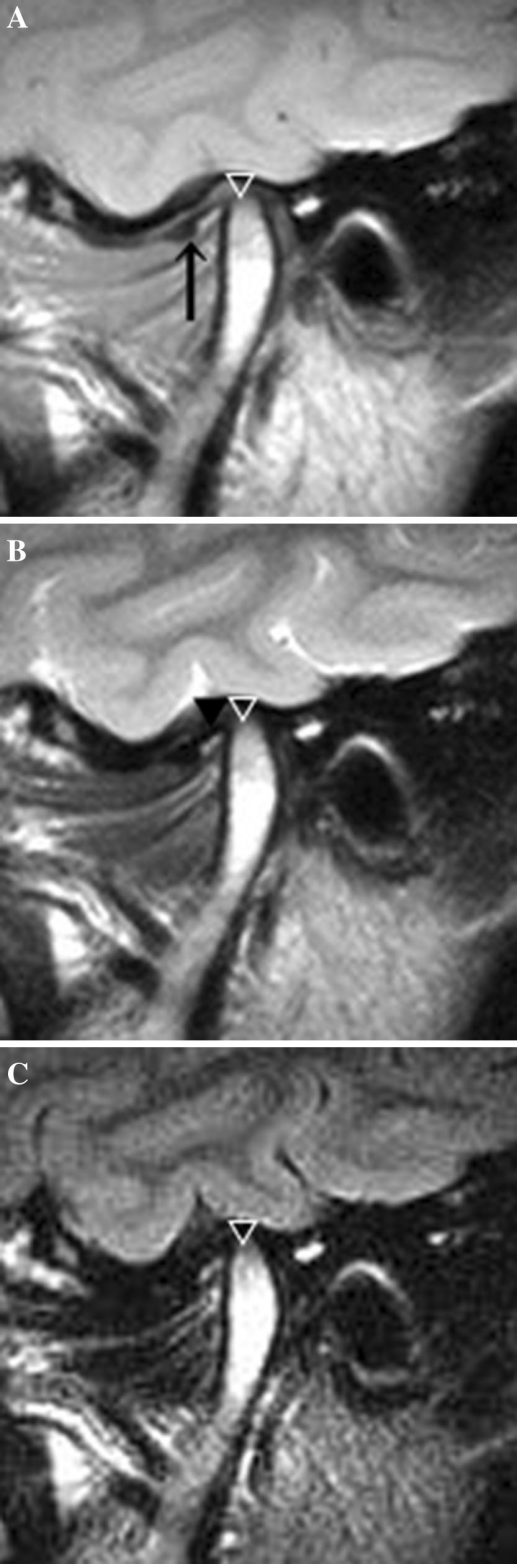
Nonpainful TMJ with disk displacement and no reduction in a 25-year-old woman. A disk (*arrow*) was medially displaced on a proton density-weighted image (a), and joint effusion (*arrowhead*) was observed in the lower joint space on a T2-weighted image (b). The SIR was 0.33 for the bone marrow of the mandibular condyle on a FLAIR image (c). No bone marrow change was apparent on any MR images (*white arrow*) (a–c)
